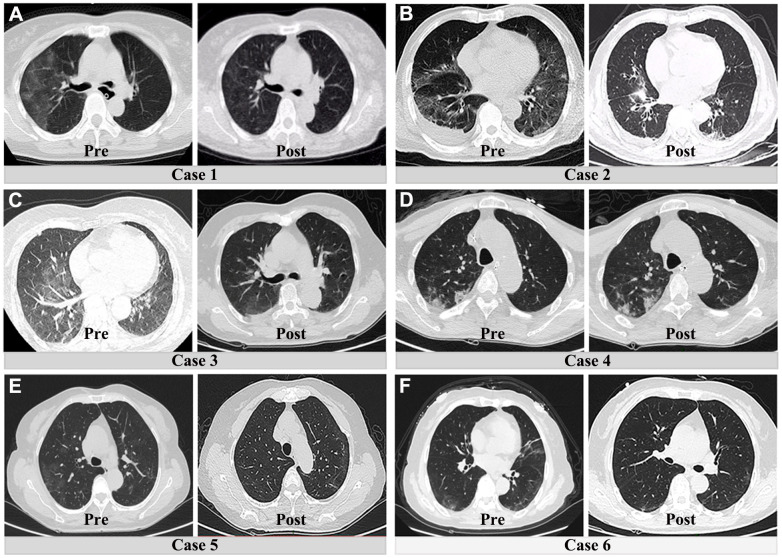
Preoperative and postoperative CT lung manifestations in six patients with both acute abdomen and COVID-19 pneumonia. (A–C) and (E, F) show the obvious resolution of pulmonary inflammation. The fourth patient had no significant change of pulmonary inflammation after surgical treatment (D).
